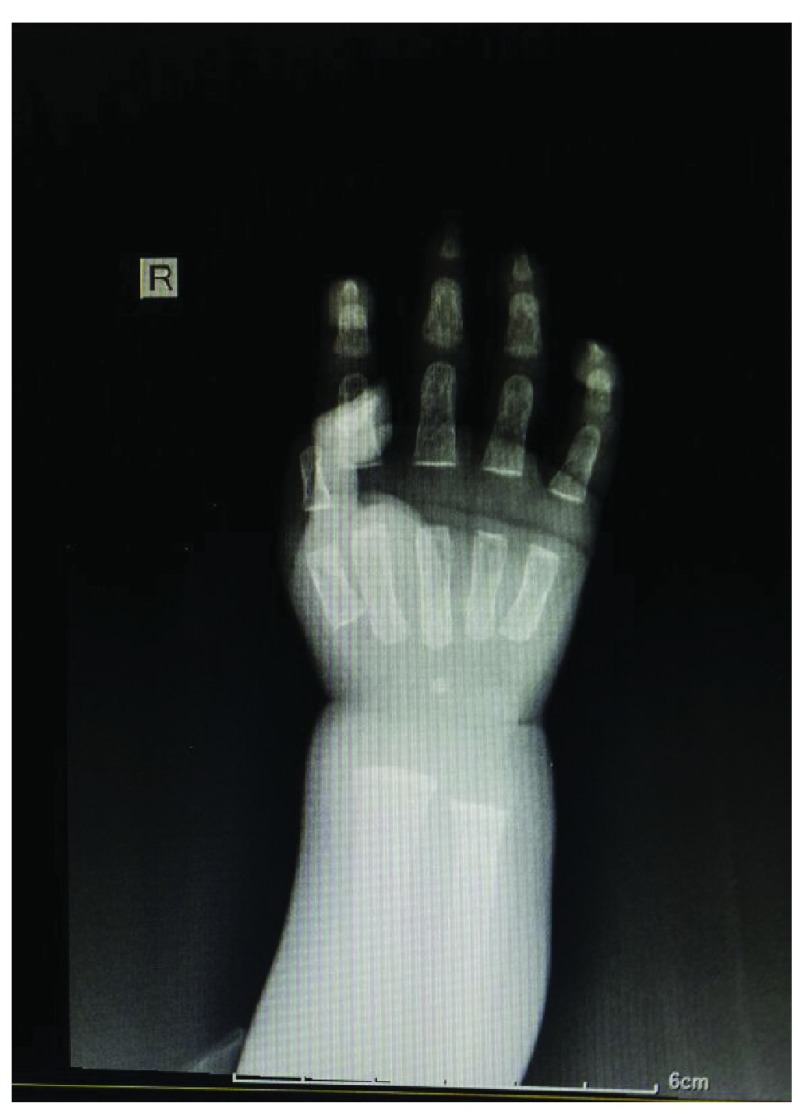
X-ray right hand showing syndactyly.
